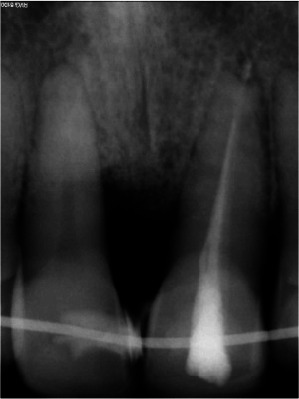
A control radiograph at 3 months post-surgery evaluating the volume stability of the added bone
